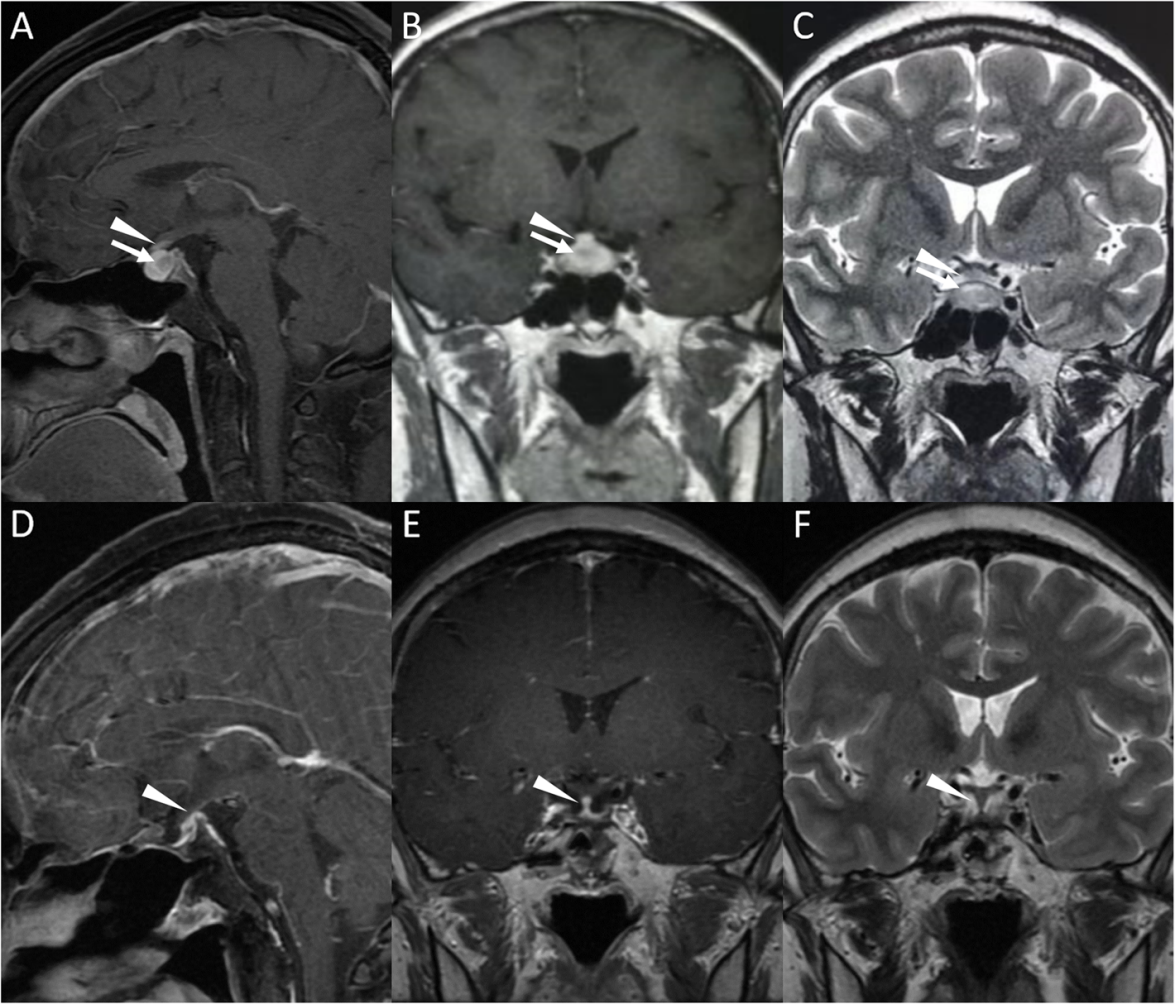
Contrast-enhanced T1-weighted and T2-weighted MR images of the lesions. a-b. Pituitary with a size of 18.6 mm × 8.2 mm × 9.9 mm displayed a contrast-enhanced signal, and a lesion with a size of 6.5 mm × 5.2 mm × 4.6 mm and a relative hypointense contrast-enhanced signal was located (arrow noted). The pituitary stalk with a size of 5.1 mm × 1.7 mm showed an isointense contrast-enhanced signal (arrowhead noted) compared with the normal pituitary stalk. c. The pituitary lesion presented a hyperintense T2-weighted signal (arrow noted), and the thickened pituitary stalk exhibited an isointense T2-weighted signal in comparison with the normal pituitary. d-f. Contrast-enhanced T1-weighted and T2-weighted MR images of the lesions 3 years after surgery. The pituitary displayed postsurgical changes with no significant hypointense contrast-enhanced signal or hyperintense T2-weighted signal, and the pituitary stalk thickness was reduced (3.0 mm × 2.0 mm, arrowhead noted) compared with pretreatment MRI
